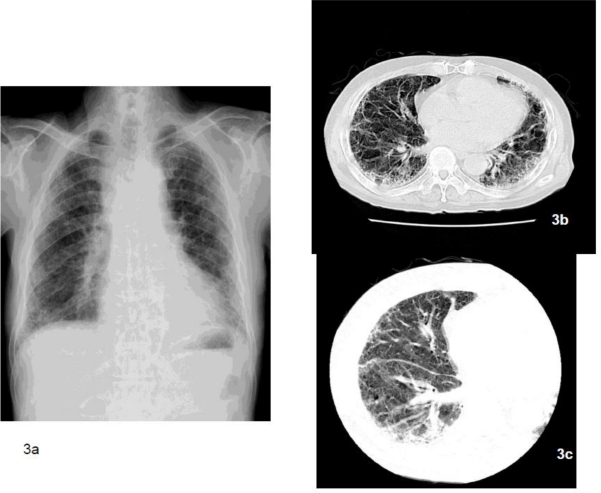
The chest x-ray (3a) indicates bilateral irregular opacity, and asbestosis was also diagnosed based on occupational asbestos exposure. However, pathologically we could not confirm the diagnosis of asbestosis in this case. The main radiological findings indicated that ground glass shadows (3b, 3c) did not accompany the honeycomb lungs. The fibrosis was observed parallel to the bronchovascular bundle. These were not inconsistent with the diagnosis of asbestosis. However, findings of fibrosis starting from the surrounding area of the bronchioles, which characteristically is the beginning of asbestosis based on pathology, were scarce and evidence of honeycomb lungs was not apparent. Asbestos bodies were sparse inside the lungs and there were 7,482 asbestos bodies/g of dry lung tissue.
